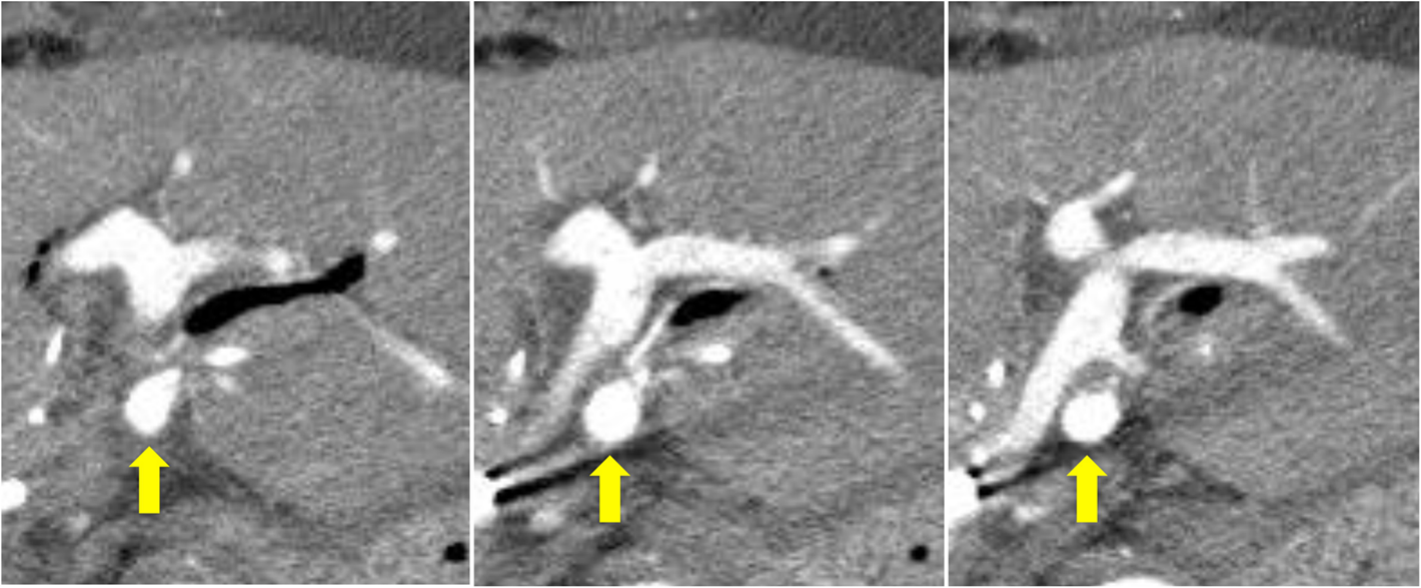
Computed tomography showing enlargement of the pseudoaneurysm (arrow) to a diameter of 10 mm on postoperative day 15
